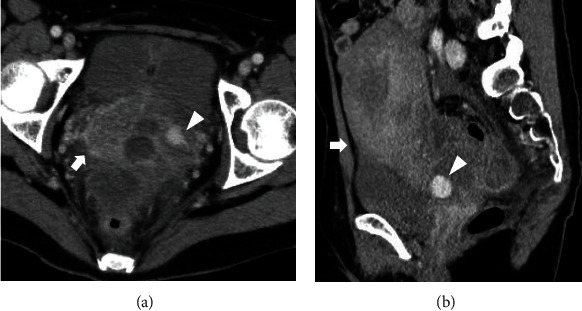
Contrast-enhanced computed tomographic images: (a) axial image and (b) sagittal image. The pseudoaneurysm, which originates from the left uterine artery, is outside of the uterine cavity. Hemoperitoneum is also observed. The arrowhead indicates the pseudoaneurysm. The arrow indicates the uterus.
